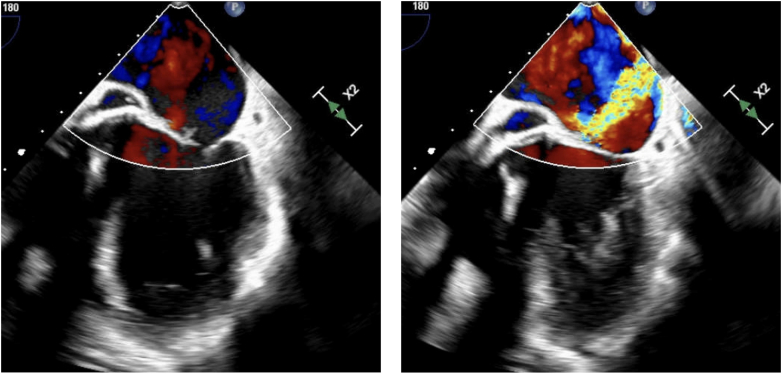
Sequential Echocardiographic Images Demonstrating Anterior Mitral Perforation Associated With Severe Mitral Regurgitation Before Repair
